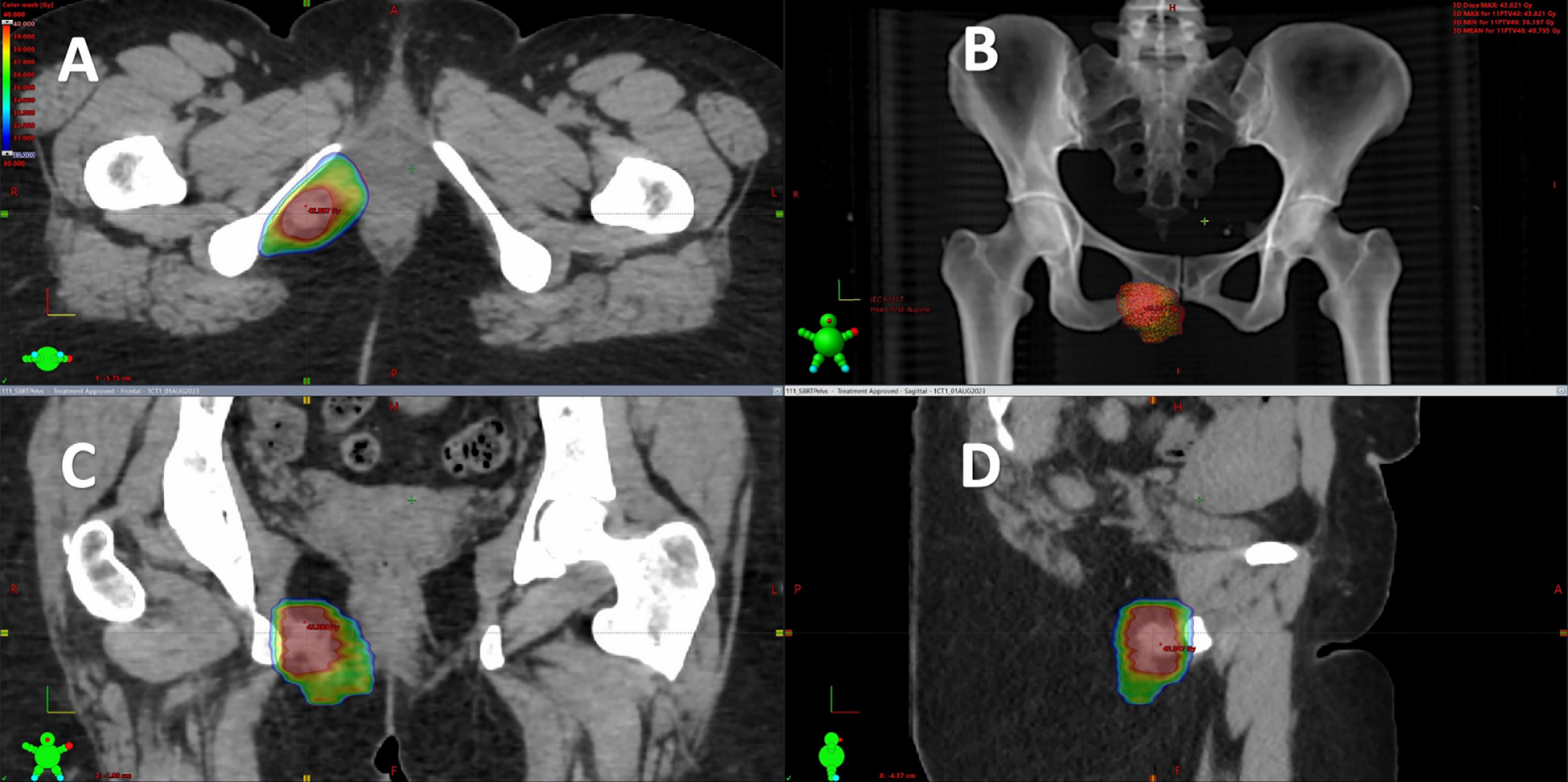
Radiotherapy plan shows stereotactic ablative radiotherapy (SABR) color wash (A, axial; B, digital reconstructed radiograph; C, coronal view; D, sagittal view). Radiotherapy software-generated 360 degrees images displayed in green Figure to orient the imaging plane.
